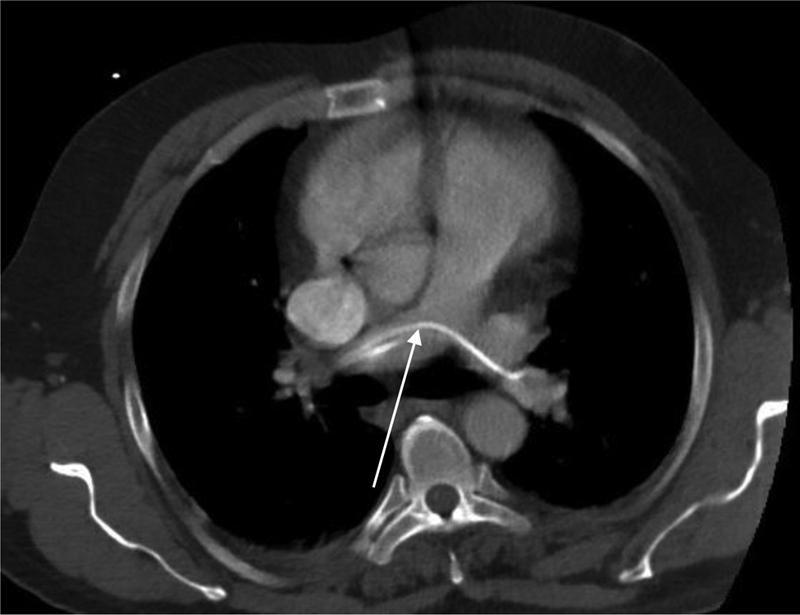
Transversal images of the CT-scan revealing the foreign body trapped in both pulmonal arteries, consistent with a CVC remnant. CT, computed tomography; CVC, Central venous catheters.
